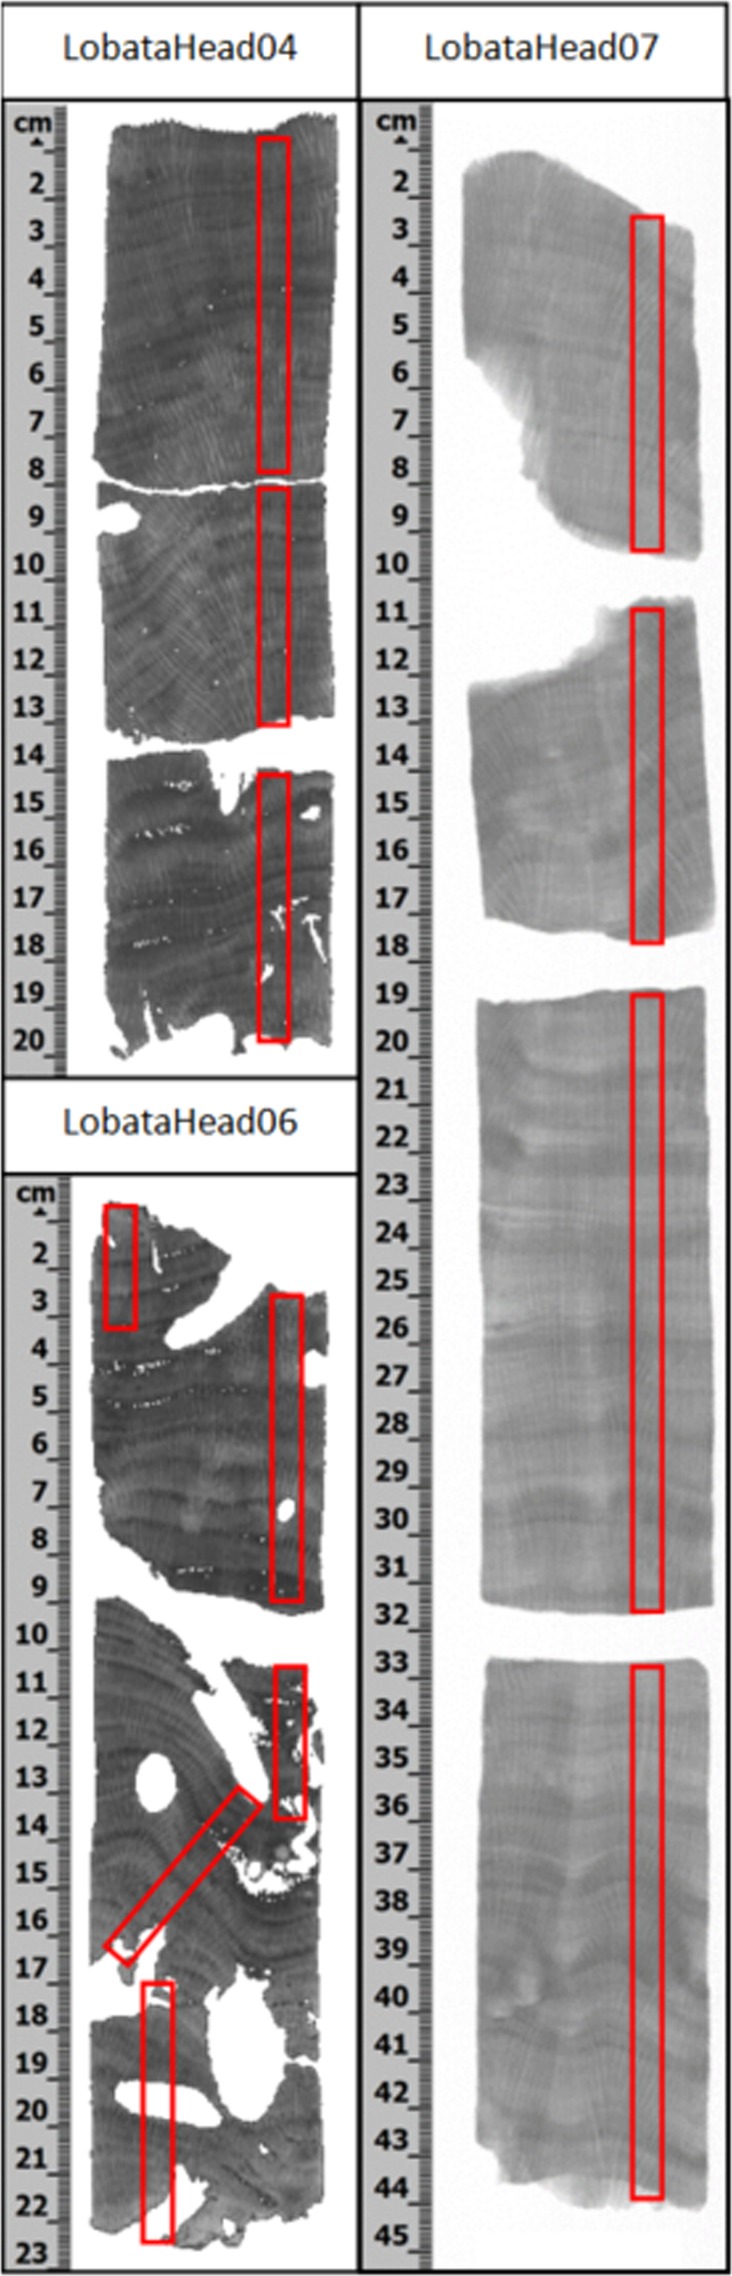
Coral core imagery. CT scan imagery of cores LobataHead04 and LobataHead06 (left), and X-Ray imagery of core LobataHead07 (right). The areas labeled in red represent the areas on the cores that were subsampled for δ^15^N analysis parallel to the coral growth axis, with a sample track width of ~0.5 cm on average.
